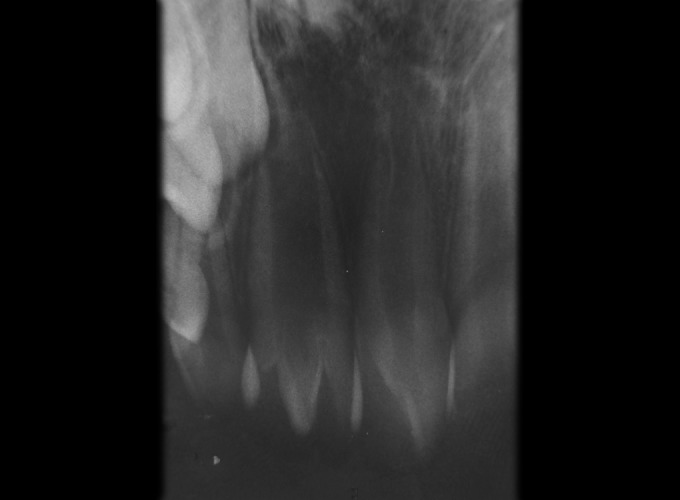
Periapical radiograph of the maxillary permanent lateral incisors
